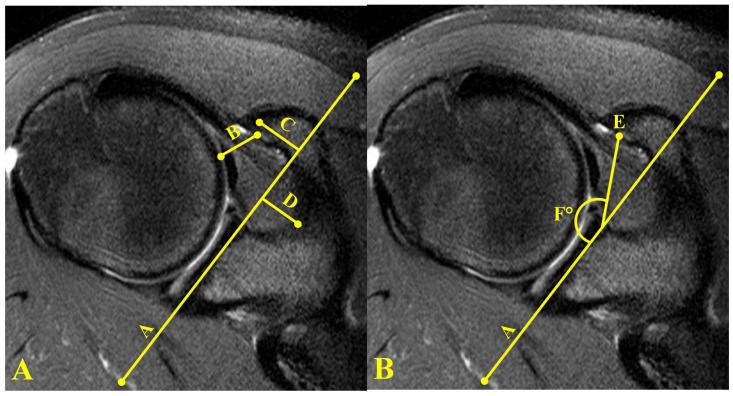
(A) Line A: the reference line drawn parallel to the glenoid on axial MRI of the shoulder. Line B: coracohumeral distance measured as the closest distance between coracoid and humerus with a line parallel to line A. Line C: coracoid overlap. Line D: coracoid recess. (B) Axial MRI of shoulder showing coracohumeral interval, Line E, and coracoglenoid angle (Angle F).
